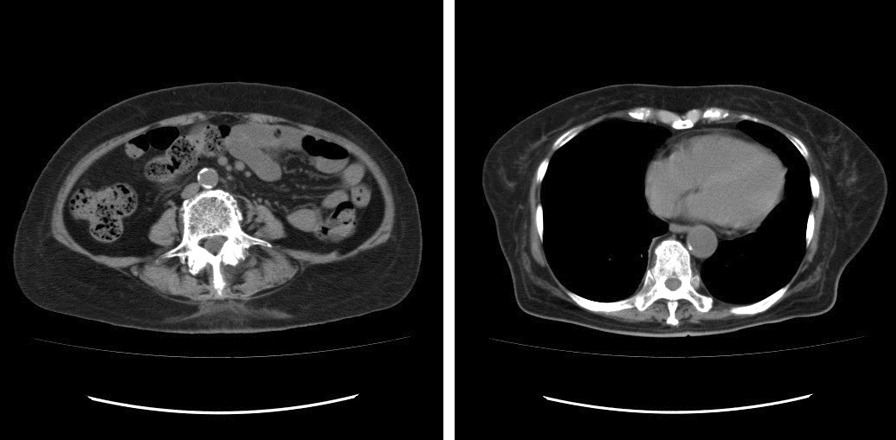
Plain computed tomography (CT) on discharge. CT revealed decreased pleural effusion and ascites and improved of wall thickening of the small intestine
